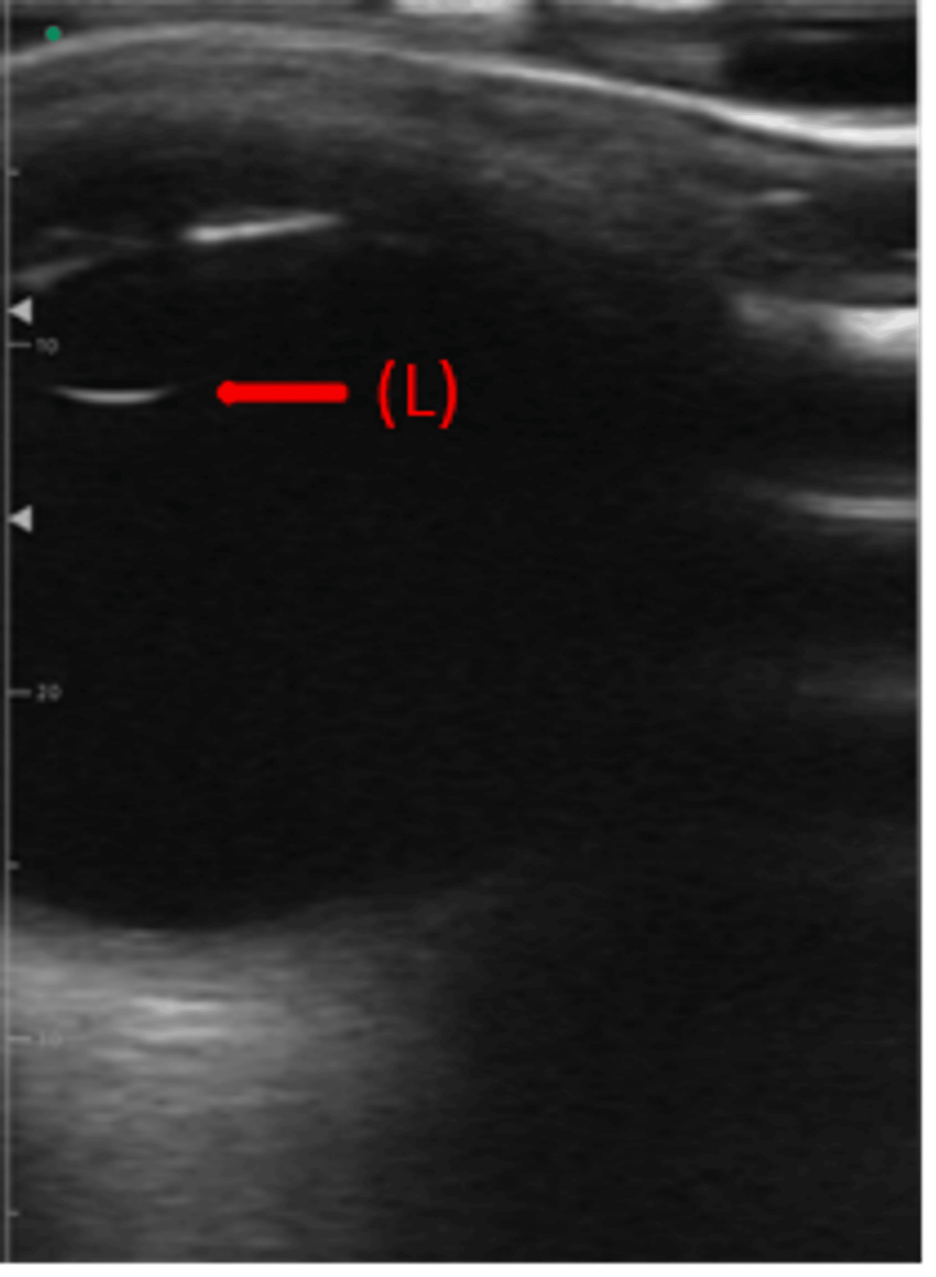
Ocular ultrasound of the patient’s left eye showing the lens (L) in a normal position without evidence of vitreous hemorrhage or structural abnormalities.
